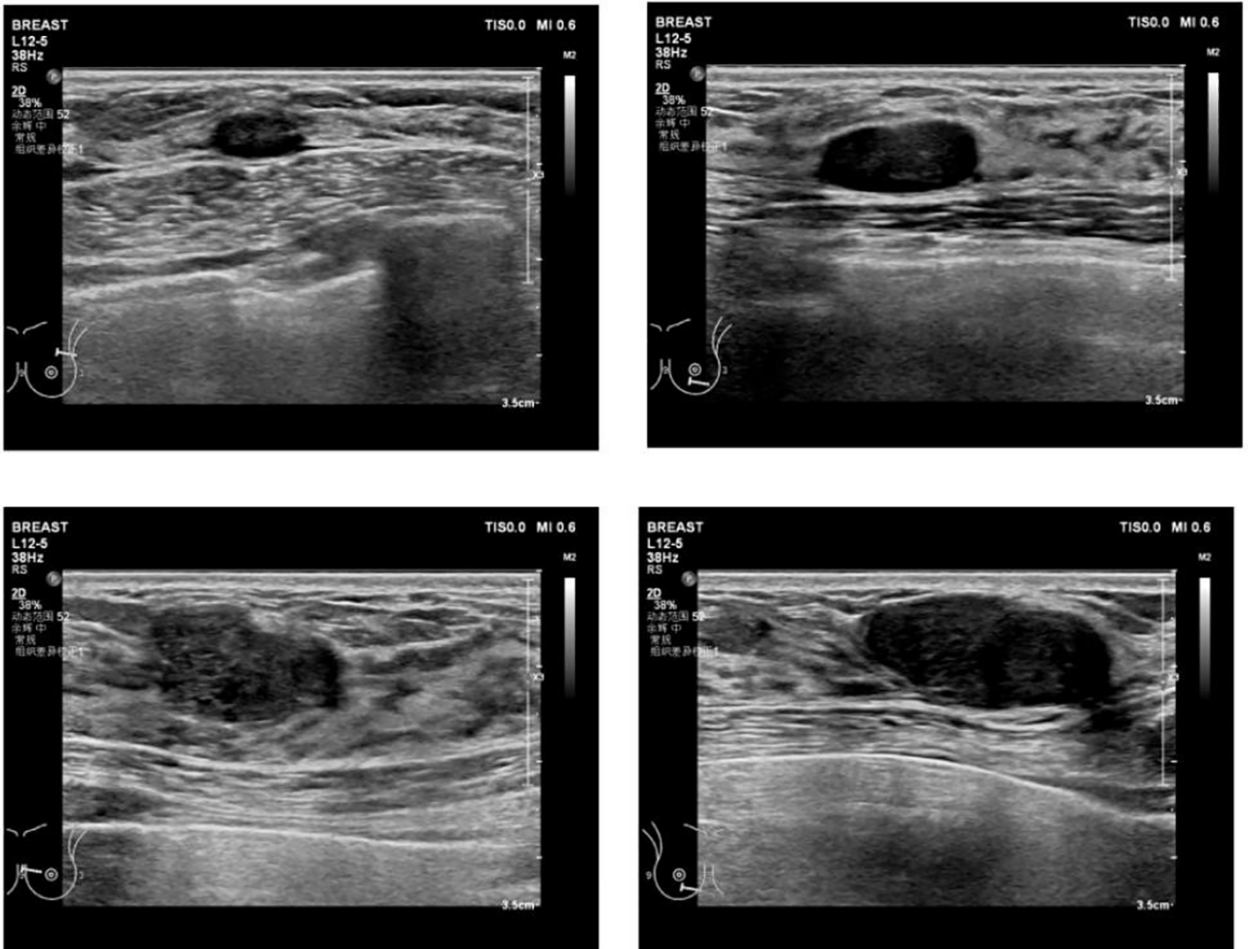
Ultrasound(US): bilateral mammary glands were irregular and flaky, local tissues were thickening, and there is structural disorder. There are several regular-shaped and well-circumscribed hypoechoic masses with envelope in her both breasts. CDFI: there was no obvious blood flow signal. Bilateral axillary lymph nodes were not palpable.
